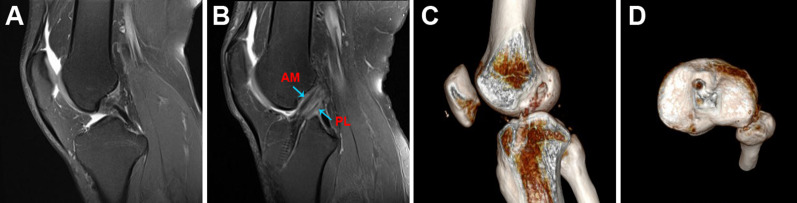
Radiological examination of STDB ACL reconstruction. A Preoperative MRI showed ACL rupture. B MRI at the last follow-up showed complete graft healing. C 3D CT showed the femoral socket and the tibial tunnel. D 3D CT showed the tibial socket
